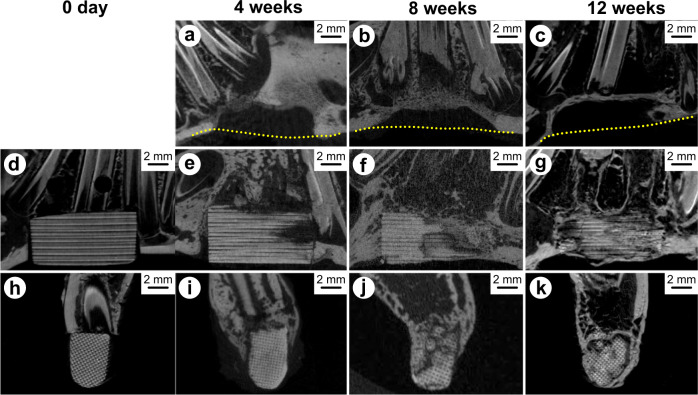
μ-CT images. The empty defect (a–c) and CO_3_Ap HCB (d–k) at day 0 (immediately after implantation) and 4, 8, and 12 weeks after implantation. The dotted lines (a–c) show the removed mandibular border. Sagittal sections (a–g) and cross-sections (h–k) of the pores
